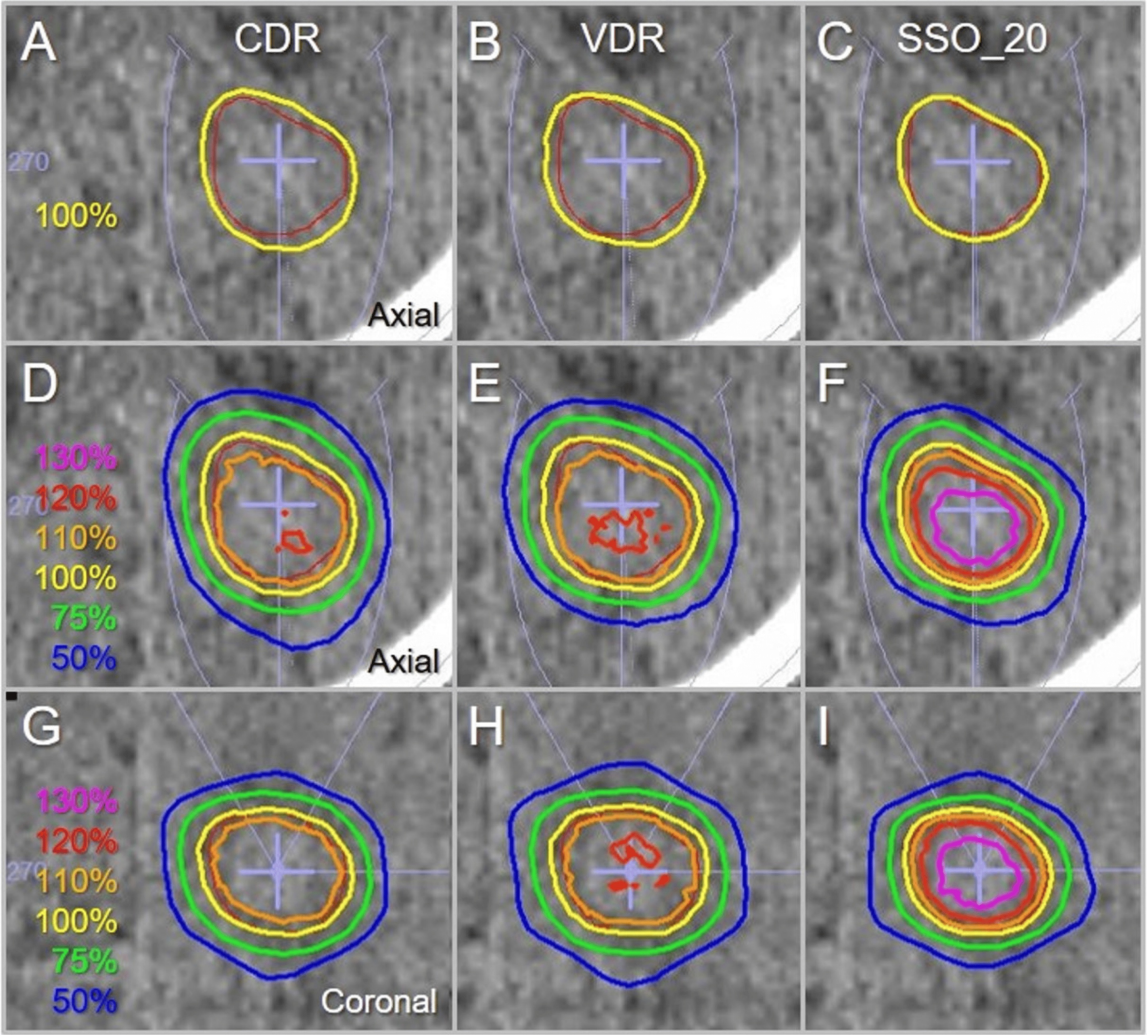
Comparison of the dose distributions for GTV of 3.20 cc. Computed tomographic images, axial (A-F); and coronal views (G-I), of a patient with a BM in the left occipital lobe (A-I) are shown. The GTV outline (red), arc arrangements (light purple), and representative isodoses in the CDR (A, D, G), VDR (B, E, H), and SSO_20 (C, F, I) are superimposed. The isodose lines are shown as the relative values to the GTV *D*_V-0.01 cc_ (100%, yellow). GTV: gross tumor volume; CDR: constant dose rate; VDR: variable dose rate; SSO_20: segment shape optimization with high-precision leaf positions value of 20; BM: brain metastasis; *D*_V-0.01 cc_: minimum dose to target volume minus 0.01 cc
